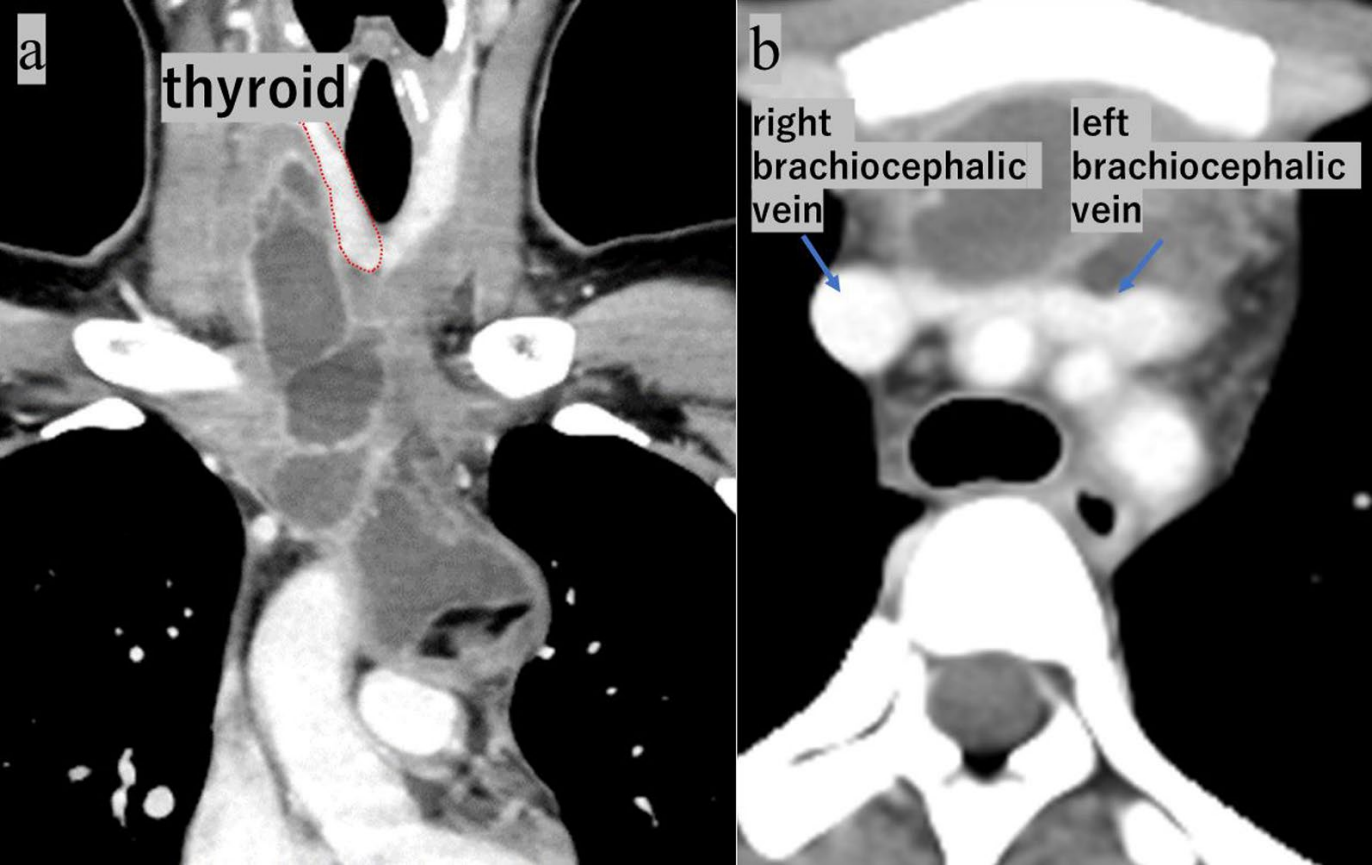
Contrast-enhanced neck and chest computed tomography revealed a multilocular cystic lesion. The lesion has extended to the right lobe of the thyroid (a) and is adjacent to both brachiocephalic veins (b)
